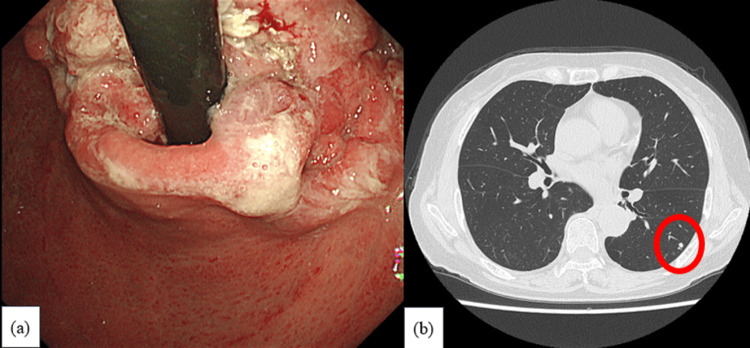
Imaging findings at inital visit (a) Esophagogastroduodenoscopy revealing gastric cardia cancer; (b) Computed tomography revealing a small metastasis in the left lower lobe (highlighted by a circle).
